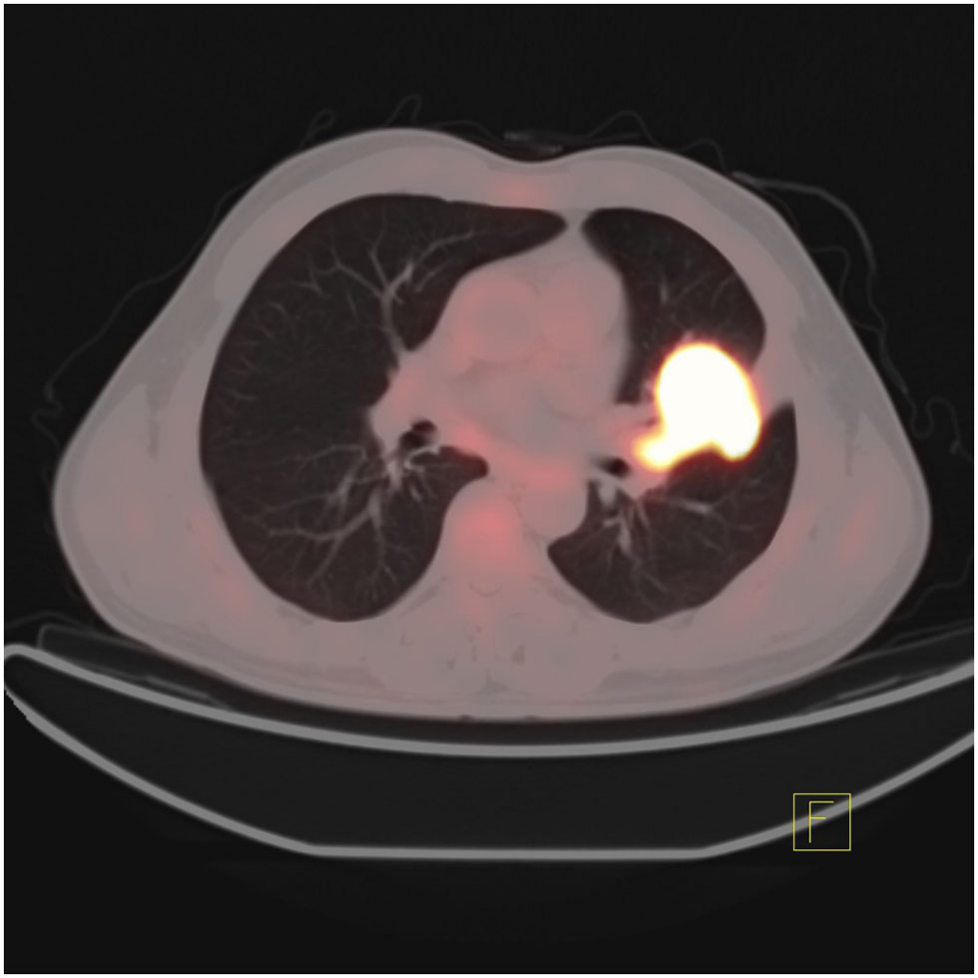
PET/CT manifestations of lung adenocarcinoma patients with EGFR mutation.
